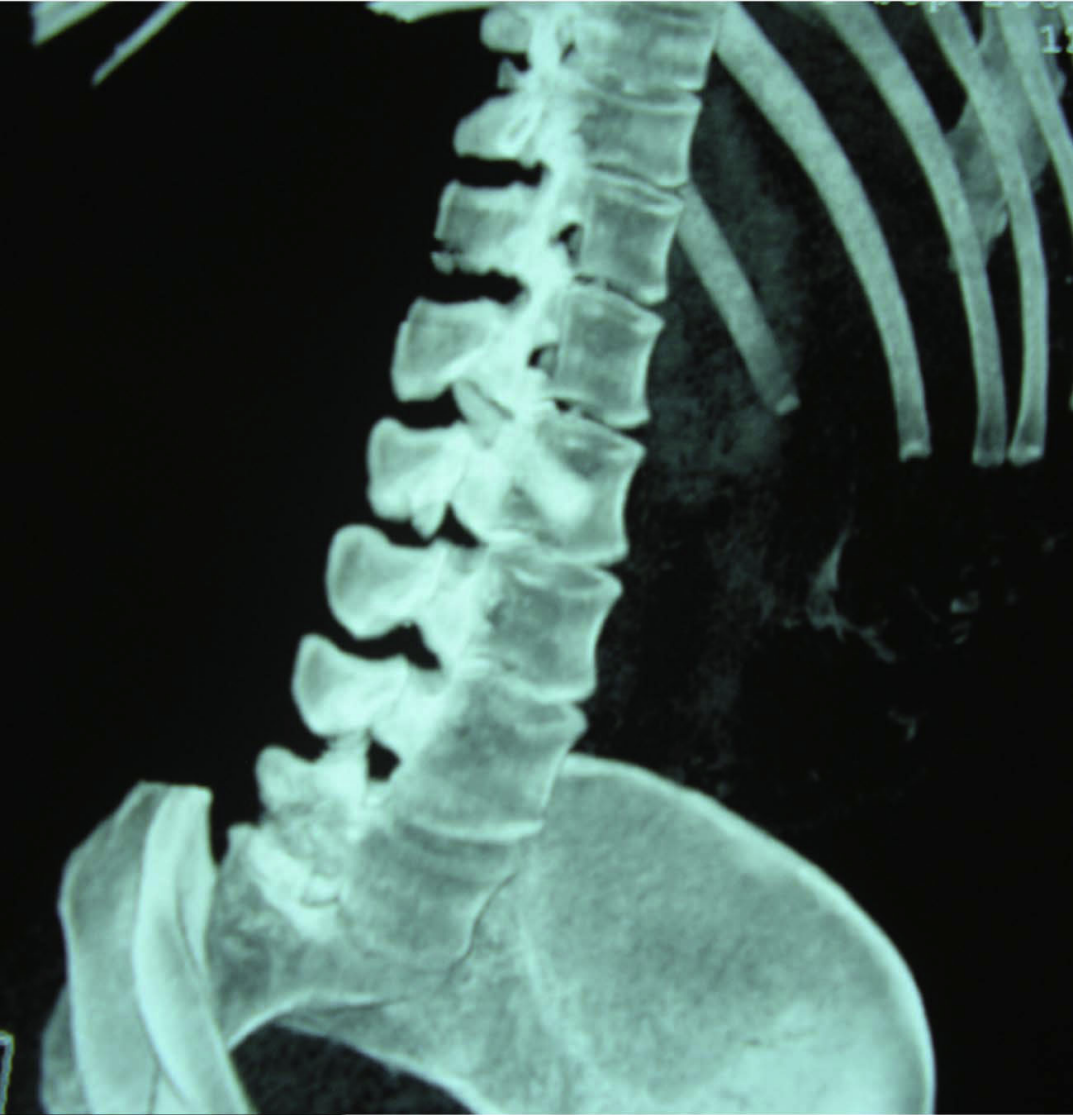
CT reconstruction image demonstrating the tip of the swordfish bill lodged within the spinal canal.
